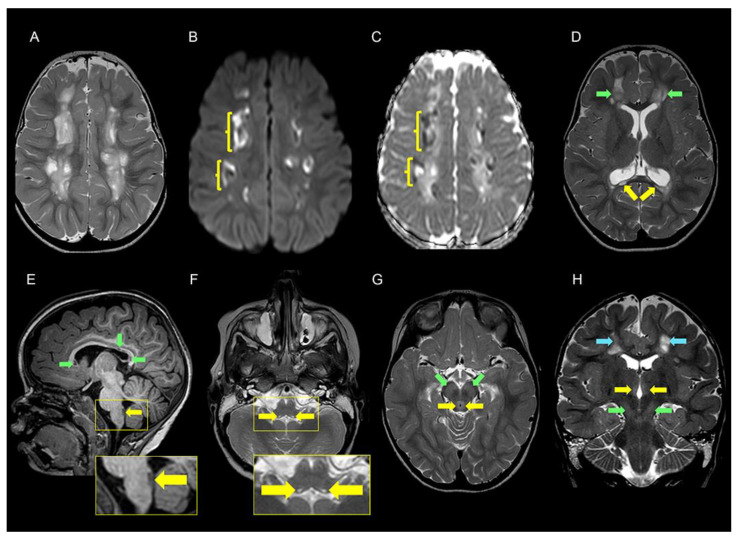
Cerebral MRI examination of the patient at the age of 2 years and 9 months. (A) T2 weighted axial image at the level of the centrum semiovale shows multiple lesions with varying degrees of high signal intensity typical of cavitary leukoencephalopathy. (B,C) Axial diffusion-weighted magnetic resonance imaging (DWI) (b1000 (B) and apparent diffusion coefficient (ADC) maps) (C) at the level of the centrum semiovale shows diffusion restriction along the margins (yellow parenthesis) of the cavitary leukoencephalopathy. (D) T2 weighted axial image at the level of corona radiata shows high signal of cavitary leukoencephalopathy in both frontal lobes (green arrows) and corpus callosum cysts (yellow arrows). (E) T1 weighted sagittal image shows thinning of the corpus callosum with low intensity foci compatible with cystic formations (green arrows). In the dorsal aspect of medulla oblongata, there is a 7 mm elongated low-signal lesion (yellow arrows). A magnification of this area is displayed in the rectangle below. (F) T2 weighted axial image at the level of the upper part of the medulla oblongata shows symmetric minute high signal changes (yellow arrows). An enlarged image of the area is shown in the rectangle below. The lesions correspond to the dorsal lesion displayed in the rectangle in E and are localized in close proximity to the dorsal motor nuclei of the vagus nerve. (G) T2 weighted axial image at the level of the mesencephalon shows high signal of periaqueductal gray matter (yellow arrows) and subtle increased signal in the substantia nigra on both sides (green arrows). (H) T2 weighted coronal image shows subtle high signal in the medial parts of both thalami (yellow arrows) and substantia nigra (green arrows) on both sides. There are also signal changes in the cerebral white matter at the level of centrum semiovale on both sides (blue arrows).
